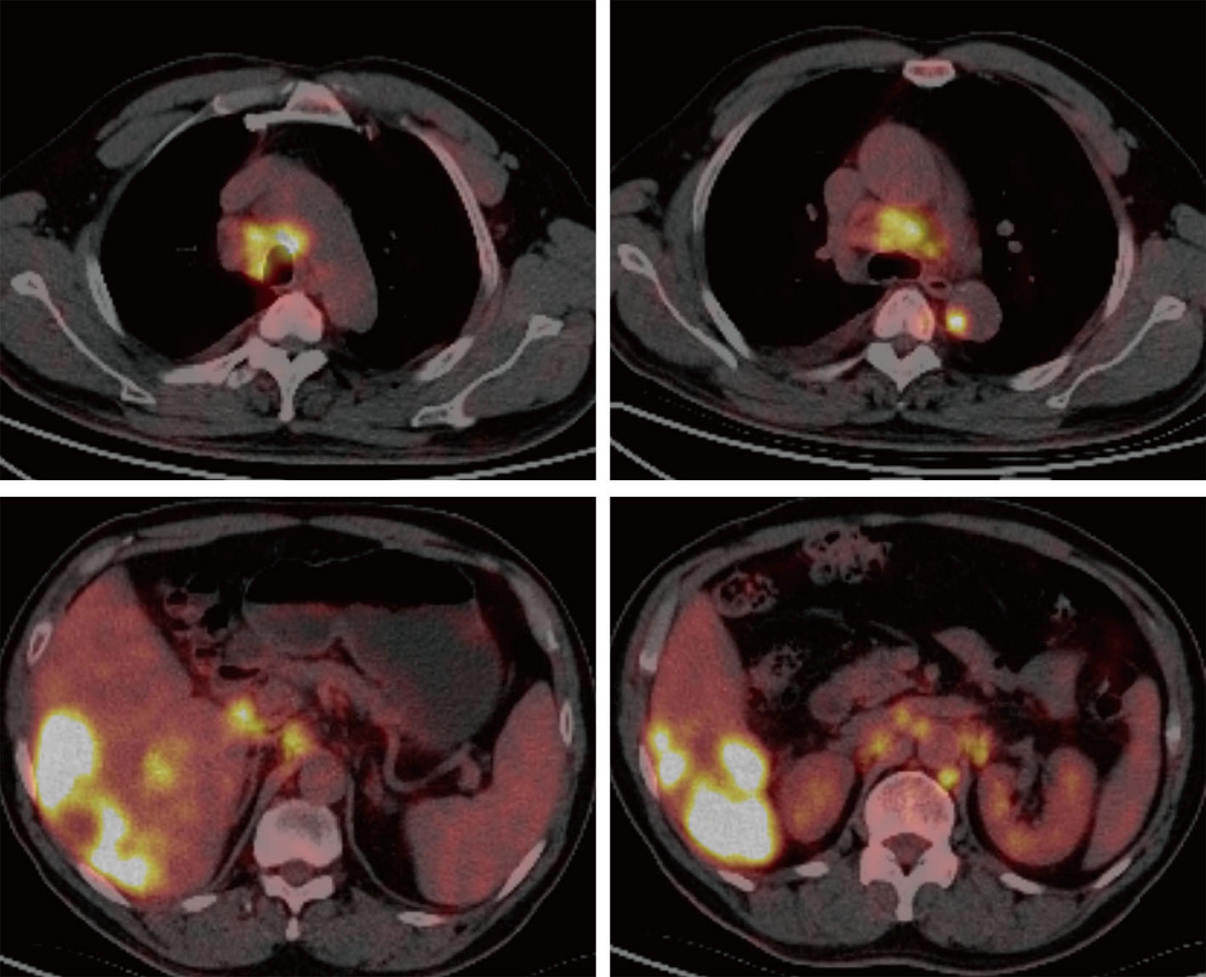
PET/CT imaging. PET/CT revealed a low-density shadow in the right liver lobe with multiple nodes in the liver, bilateral supraclavicular and subclavian lymph nodes, mediastinal lymph nodes, lymph nodes on the left side of the spine, celiac lymph nodes, retroperitoneal lymph nodes, and vertebral body metastases.
